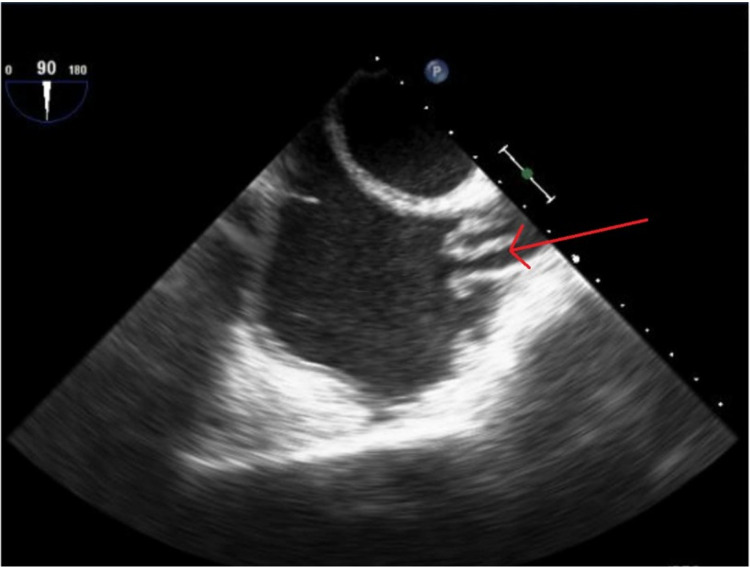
Endovascular thrombotic cast with mobile elements roughly 1.1 x 2.7 cm noted in the SVC during a TEE SVC: superior vena cava; TEE: transesophageal echocardiogram
